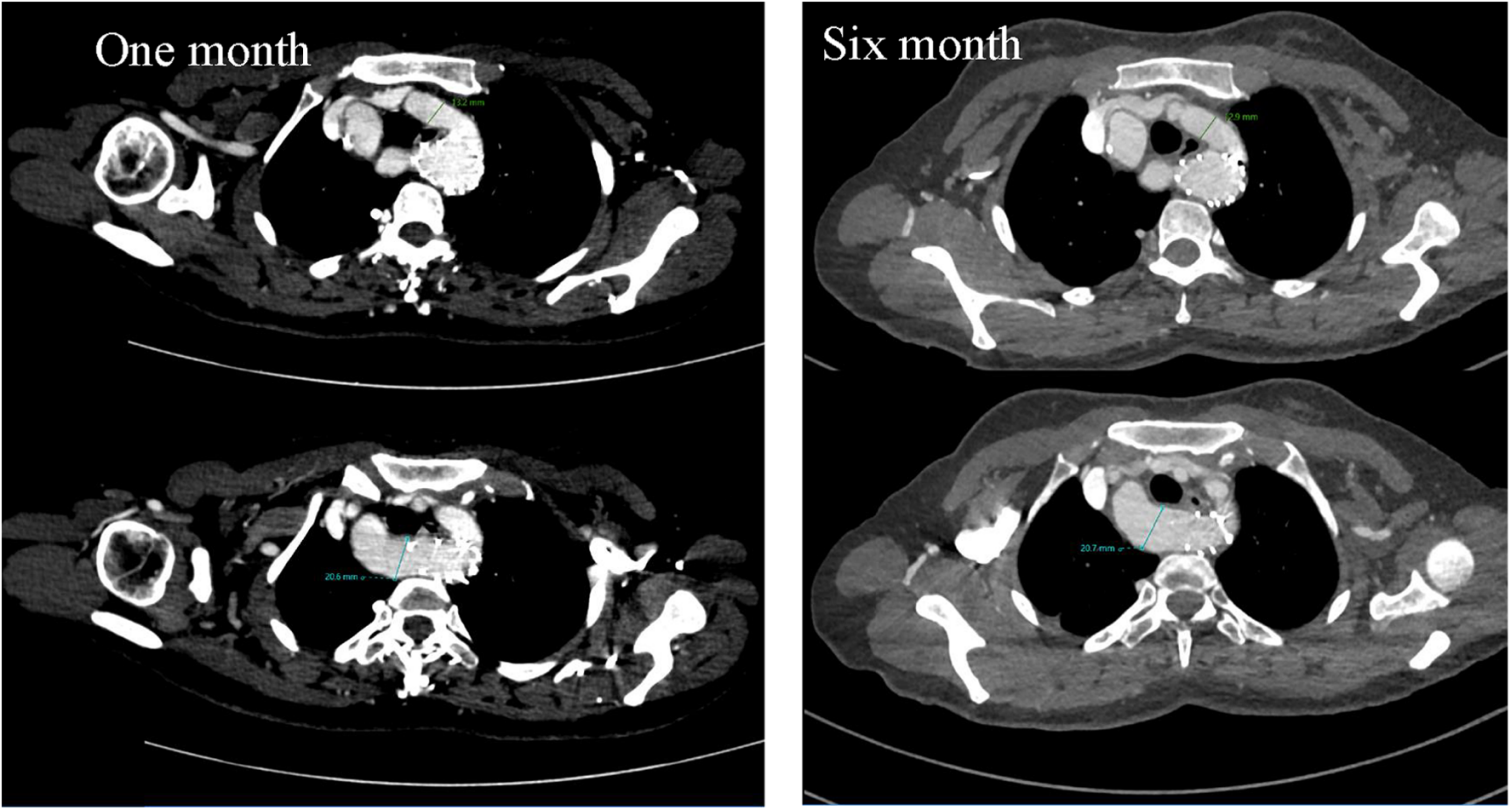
Cross-sectional images of postoperative CTA at one month and six months suggested no significant changes in dominant and non-dominant arch diameters and no obvious change as preoperatively.
